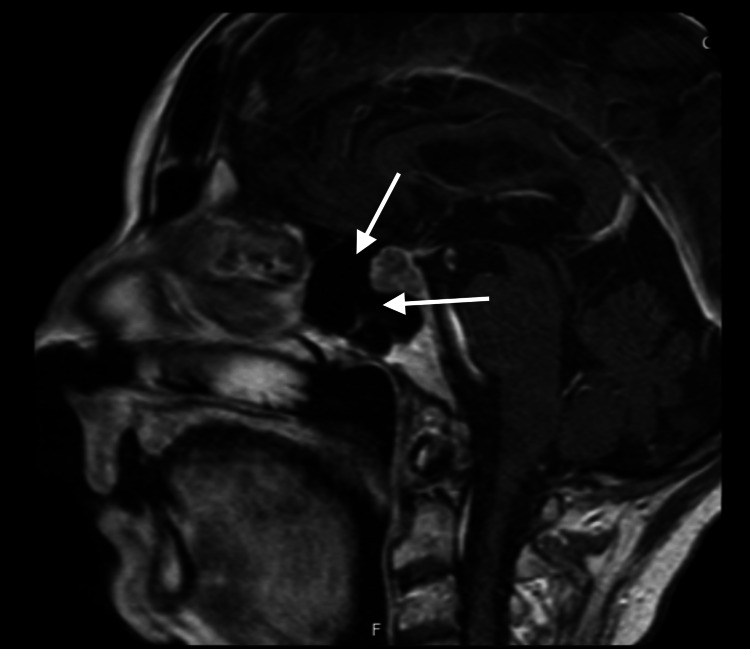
MRI Brain (Coronal section) MRI head showed a macroadenoma (pituitary macroadenoma). It is abutting optic chiasma. It measures length 13.7 mm x height 12.8 mm x breadth 18 mm. The pituitary stalk is thickened. The tuber cinereum is imaged normally.
